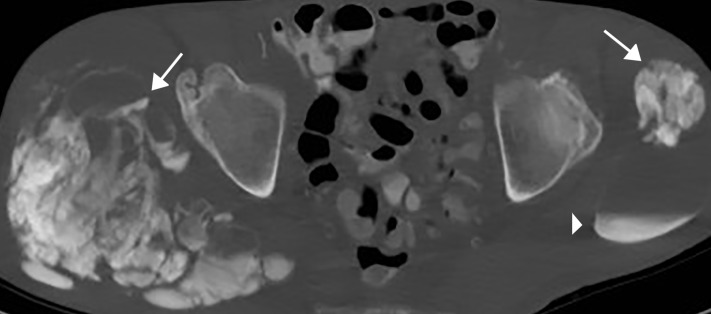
Bilateral hip CT Axial CT image at the level of the hips demonstrates bilateral, dense, multilobulated periarticular masses (arrows). Sedimentation sign (arrowhead).
